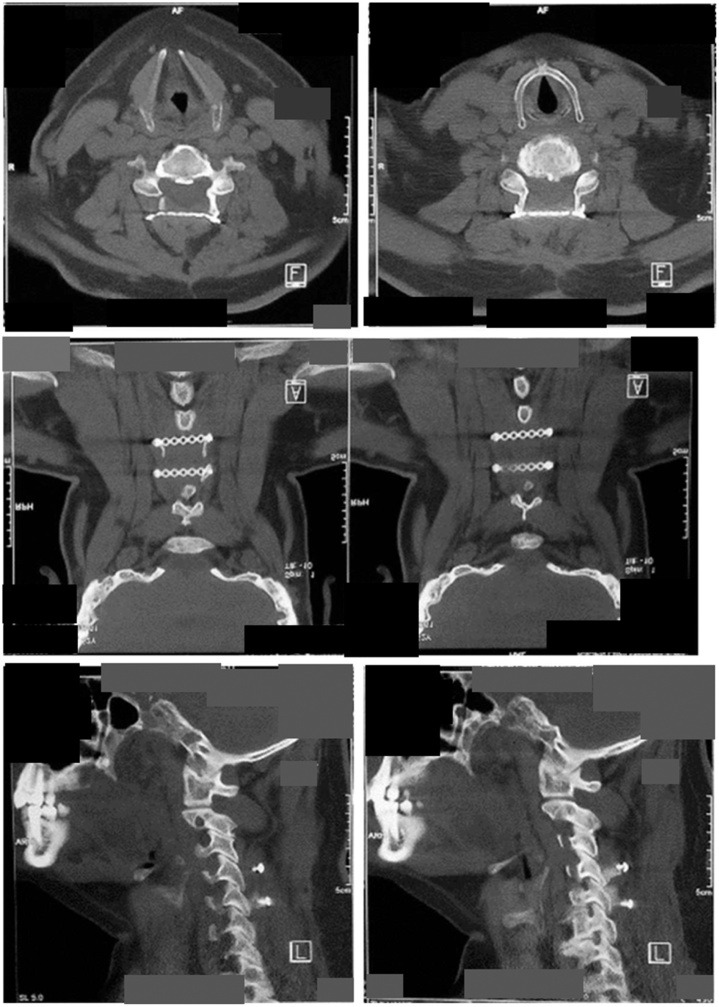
Post-operative cervical spine computed tomography scan.
